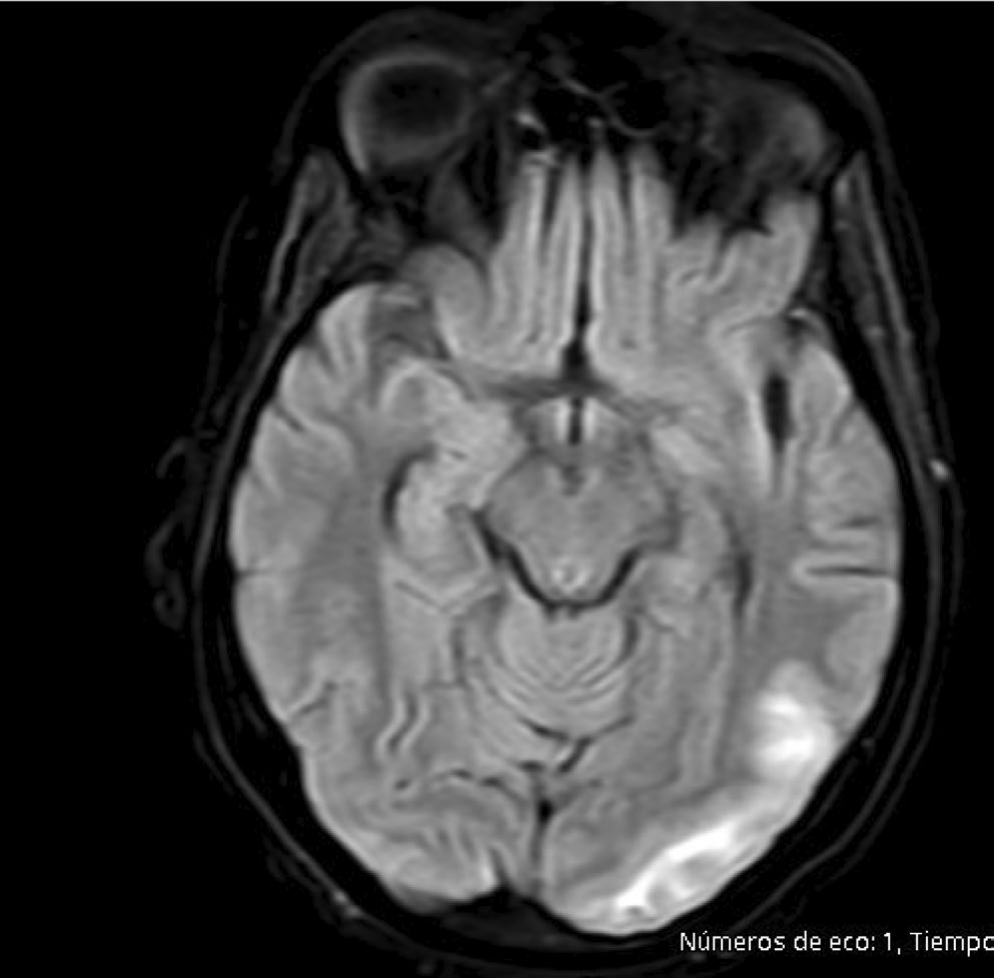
FIGURE 3 Left temporoocipital hypersignal, FLAIR.
